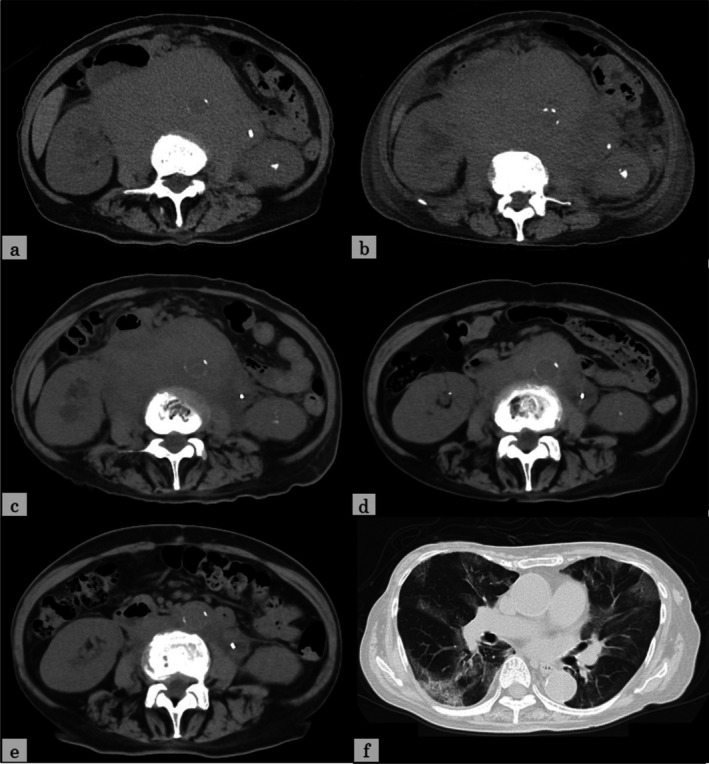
Computed tomography (CT) images. The bulky abdominal tumor shows a slight temporary increase after starting epcoritamab, but then it shows a marked decrease (a: before epcoritamab, b: 4 weeks after starting epcoritamab, c: 8 weeks after starting epcoritamab, d: 12 weeks after starting epcoritamab, e: 5 months after starting epcoritamab). COVID‐19 pneumonia develops just before the final dose of the fourth cycle of epcoritamab (f).
